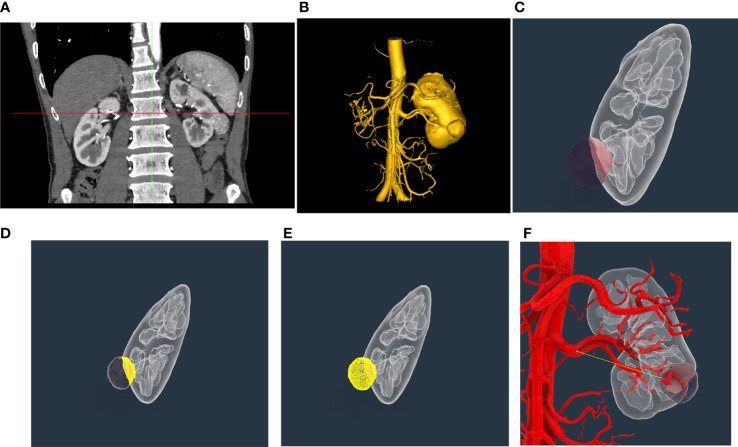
Representative three-dimensional (3D) reconstruction of enhanced abdominal CT and measurement of indicators of tumor mass. (A) Representative enhanced abdominal CT scan image of a kidney cancer patient. (B) Three-dimensional reconstruction of the kidney based on enhanced abdominal CT by Mimics 24. (C) Exhibition of tumor mass in Rhinoceros 3D; (D) Automatic calculation of tumor bed area using Rhinoceros 3D. (E) Automatic calculation of surface area and volume of tumor mass using Rhinoceros 3D. (F) Manual measure of the distance from the tumor to the first bifurcation of the renal artery in the tumor tissue using Rhinoceros 3D.
